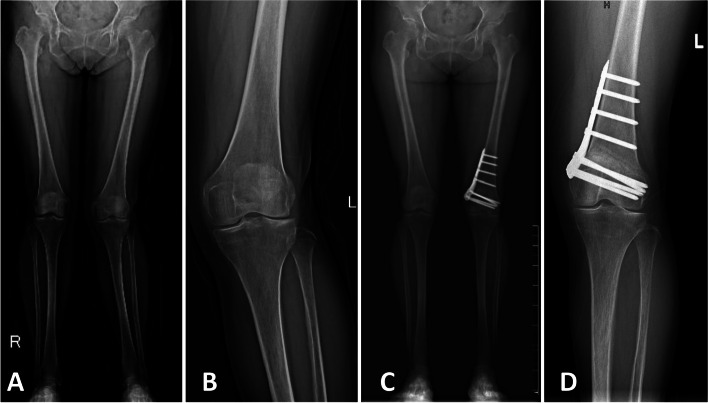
Preoperative (A) and postoperative (C) full-length weight-bearing radiographs of the lower limbs, and preoperative (B) and postoperative (D) anterior–posterior view radiographs of the affected knee of patients with isolated valgus deformity and lateral compartmental osteoarthritis
